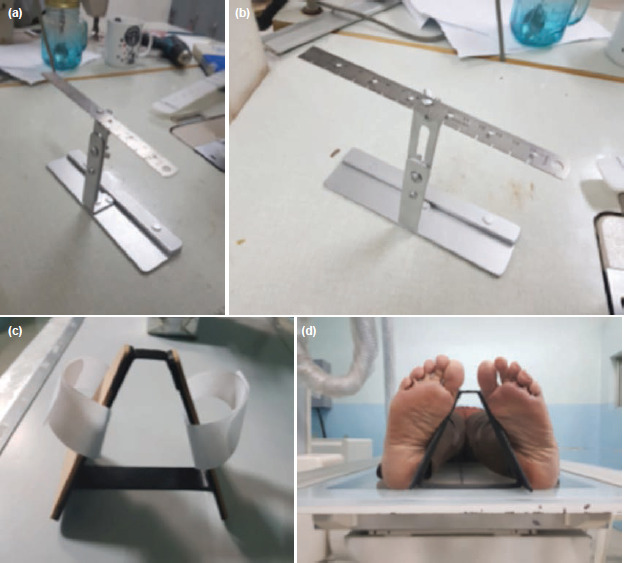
(a, b) Radiographic ruler with adjustable height, (c, d) 20° internal rotation foot angle guide with base at 20cm apart.
